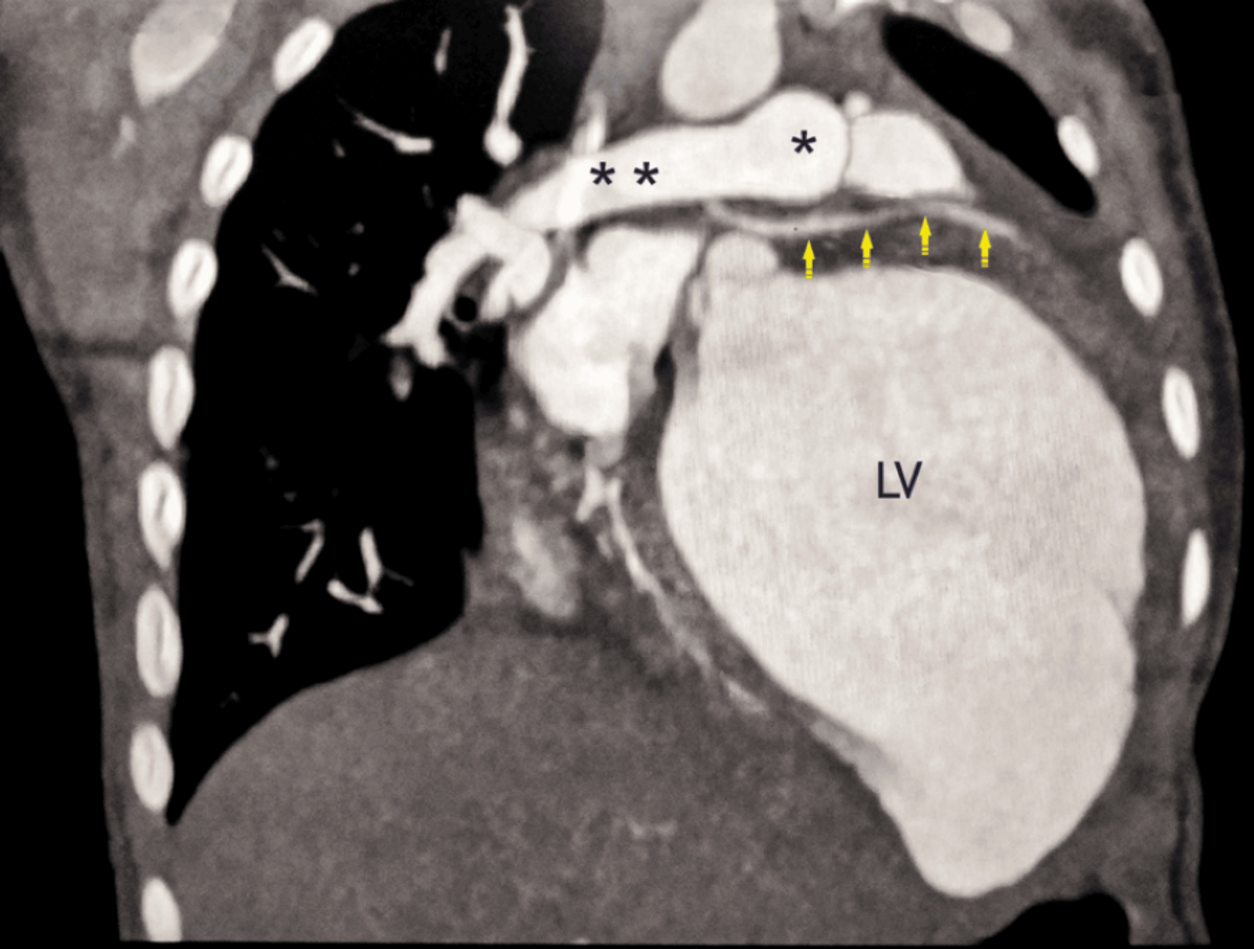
CT angiography with oblique coronal maximum intensity projection (MIP) reconstruction showing the abnormal origin of the LCA from the underside of the RPA (ALCARPA, yellow arrows) with a dilated left ventricle (LV). , RPA, right pulmonary artery; *, MPA, main pulmonary artery; CT, computed tomography Image Credits: Dr. Sachin Talwar
